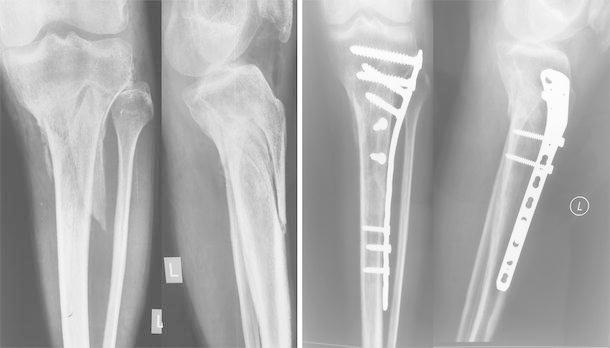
Preoperative and postoperative radiographs of a patient treated with plating
